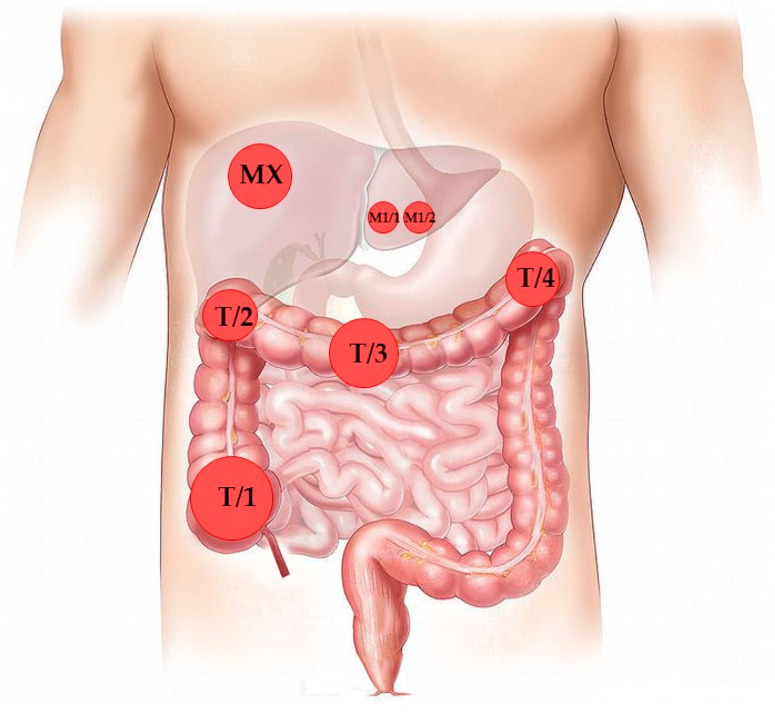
Anatomical localization of the individual primary tumors of the upper colon and of the liver metastases known at the time of the surgery. MX represents liver metastasis described by preoperative CT scan examination but removed later as M2 (hepatic VIII. segment).
